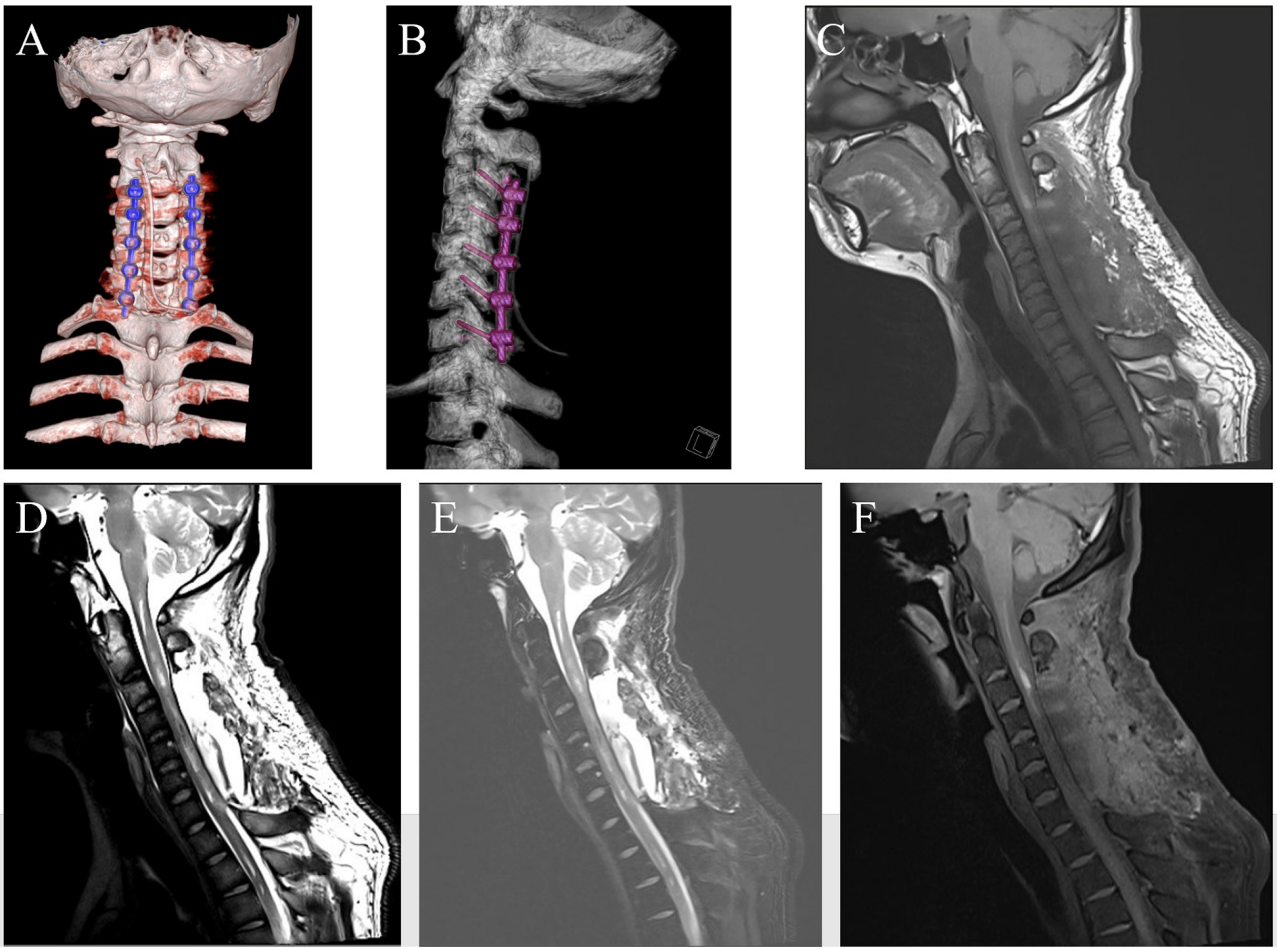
Postoperative follow-up imaging 1. (A,B) On postoperative day 1, cervical CT was reviewed. (C–F) At 1 week postoperatively, cervical MRI was reviewed.
